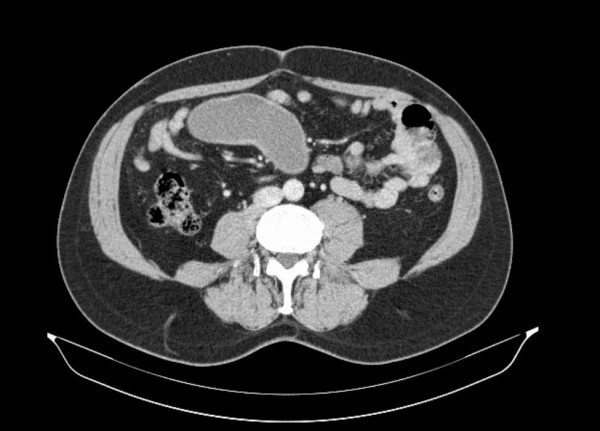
Computed tomography scan showing a low-density cystic mass (4 × 10 cm) with an enhanced margin in the mid-abdomen.
